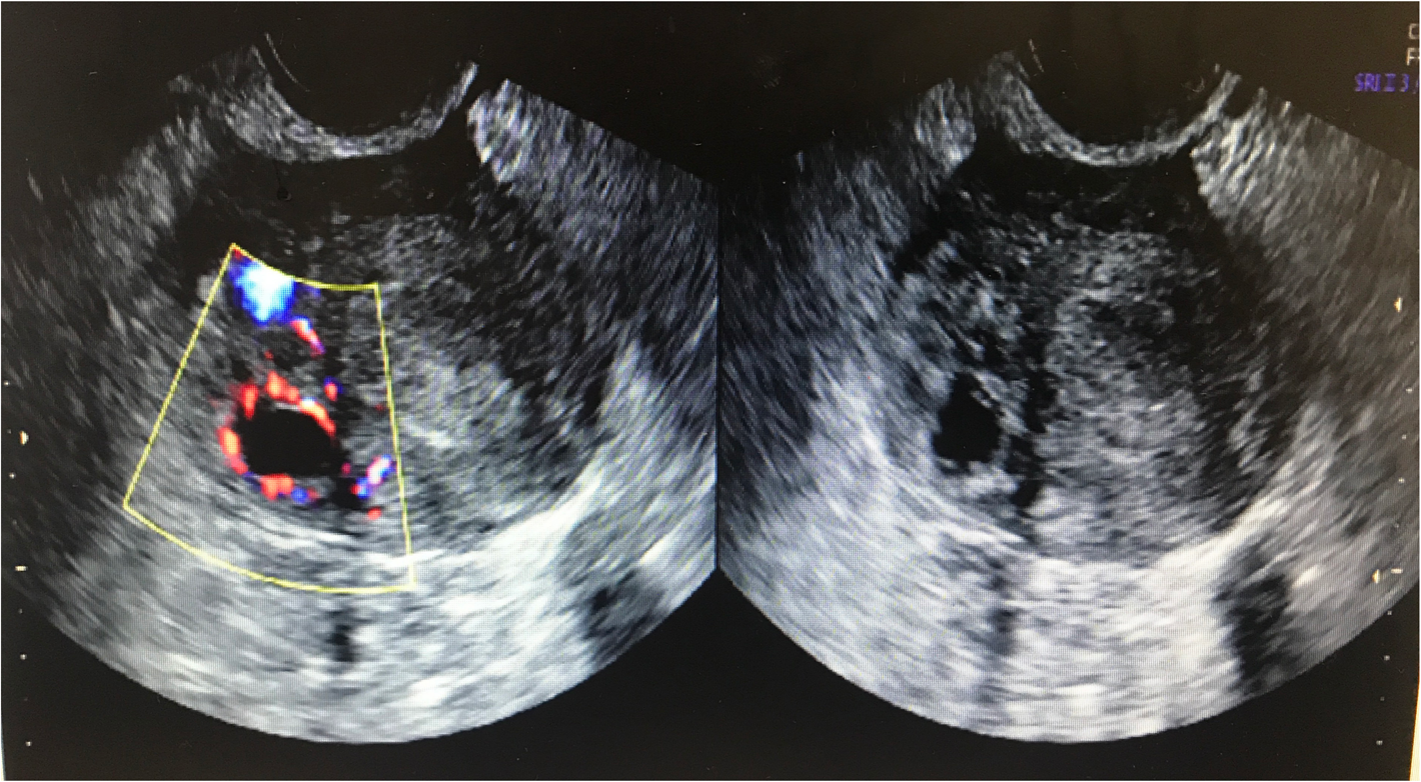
Transvaginal ultrasonography showed the gestation located in the right uterus cornu
